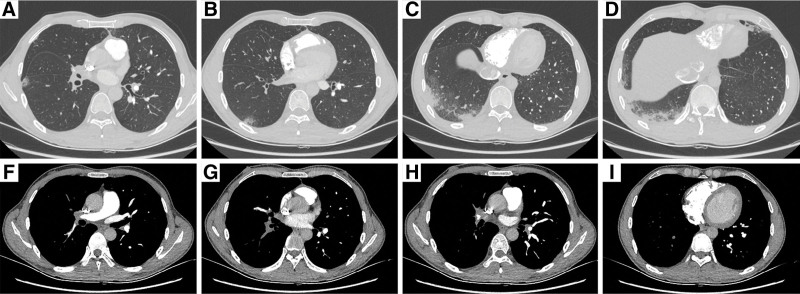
CT and CTPA imaging results of the lung. (A–D) The pulmonary infarction focus. (E) Wall thickening and lumen stenosis of the main right pulmonary artery. (F–H) Right middle lobe and lower lobe pulmonary artery branch occlusion. CT = computed tomography, CTPA = CT pulmonary angiogram.
